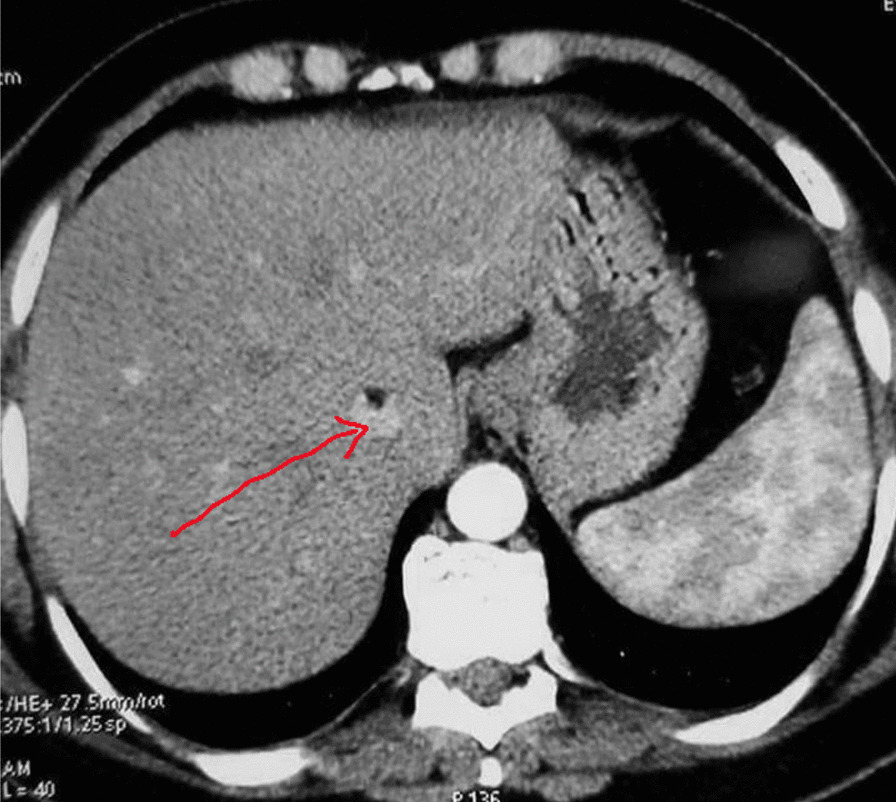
Computed tomography scan showing thrombus in the vena cava (arrow)
